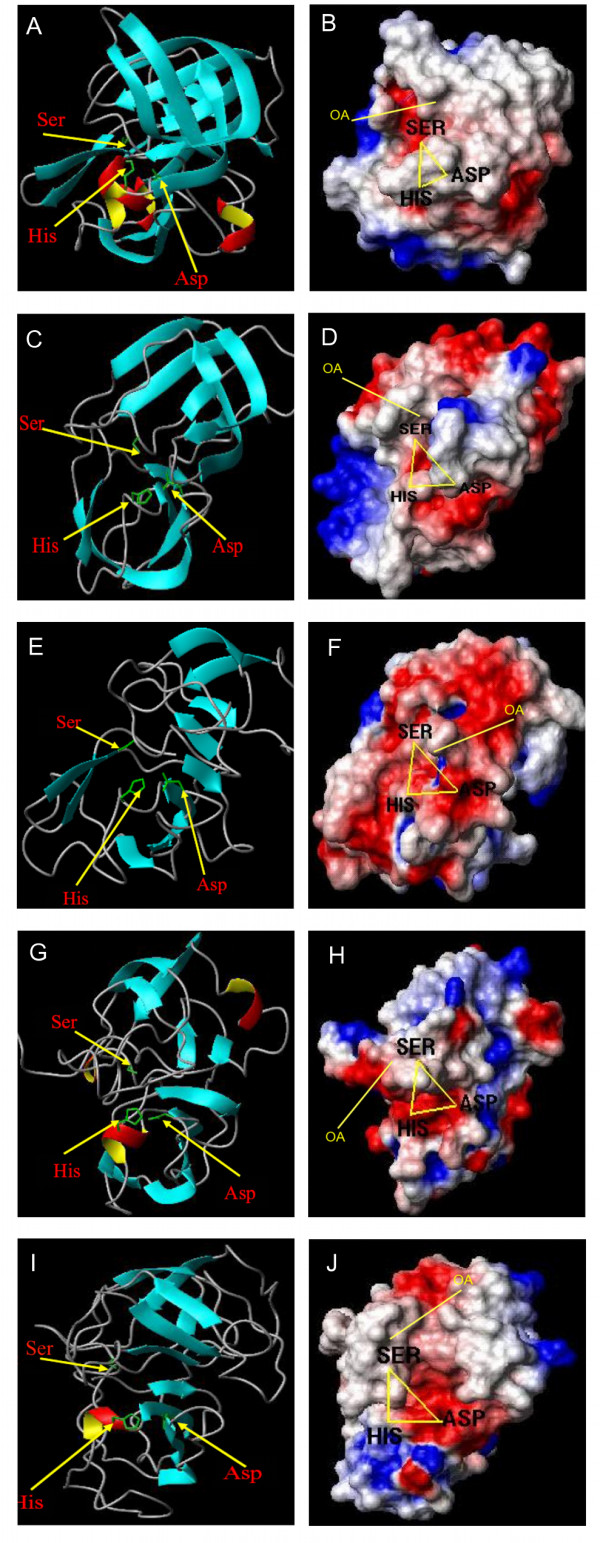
A representative X-ray PA protease structure from*Streptomyces griseus*and modeled PA protease structures from*Plasmodium falciparum, Pyrococcus furiosus, Neurospora crassa and Arabidopsis thaliana*. Ribbon models of *S. griseus*, 1SGC (A), *P. falciparum* (C)*, P. furiosus* (E)*, N. crassa* (G) *and A. thaliana* (I) PA protease structures show β-sheets with an arrow directed to the C-terminus (light blue), α-helices (red and yellow), turn/loops (gray), and catalytic triad residue side chains (green sticks). Surface electrostatic potential model of *S. griseus*, 1SGC (B), *P. falciparum* (D)*, P. furiosus* (F)*, N. crassa* (H) *and A. thaliana* (J) PA protease structures show electronegative (red), electropositive (blue) and electroneutral (white) amino acid side chains. The estimated position of the oxyanion hole (OA) is also indicated.
